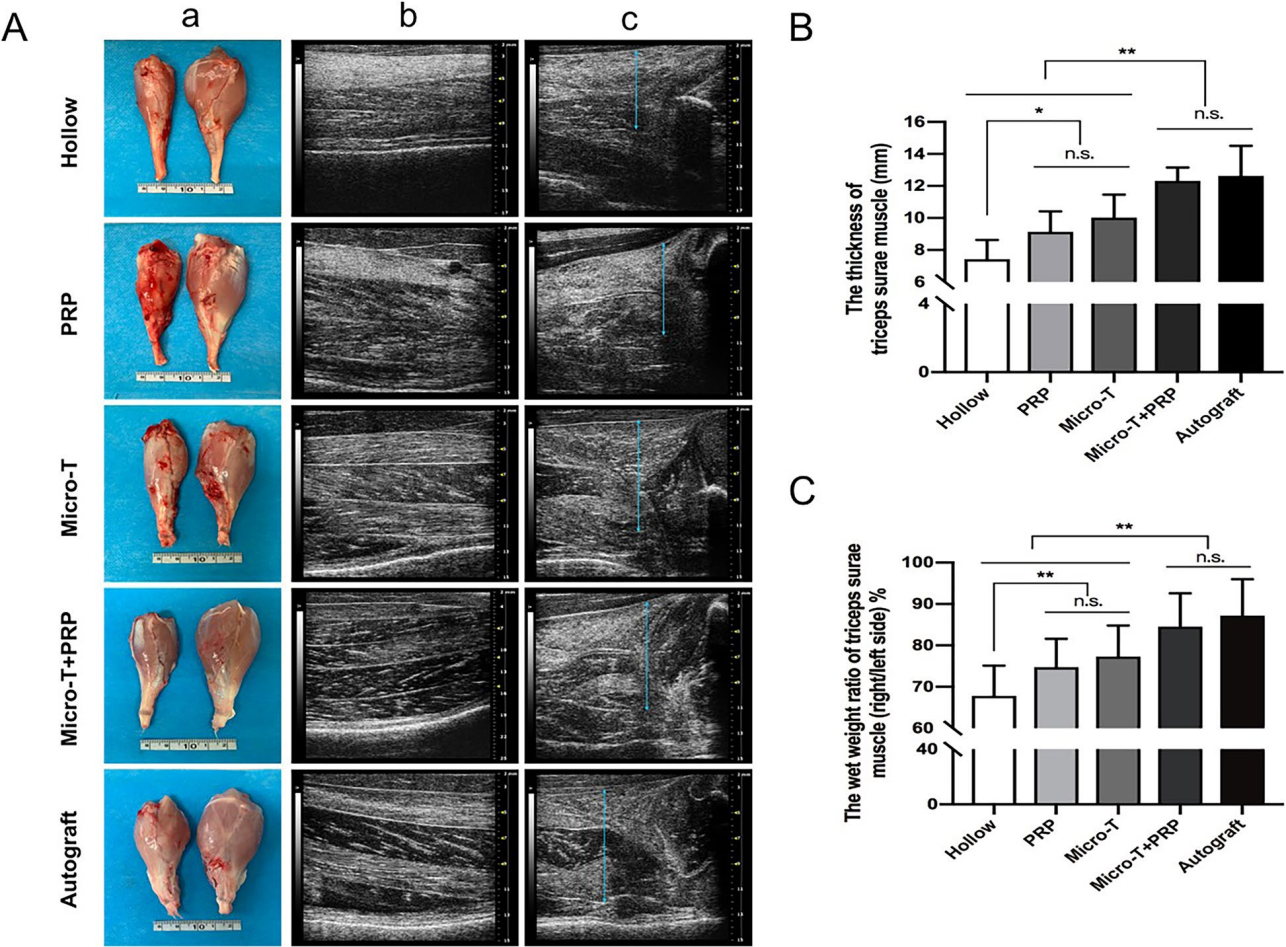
Macroscopic evaluation and high-frequency ultrasound evaluation of the targeted muscle. (A) Images of the targeted muscle. (a) The gross image; (b) The triceps surae muscle in the longitudinal section; (c) The thickness of the triceps surae muscle in the transverse scan. (B) Comparison of the thickness of triceps surae muscle in each group. (C) Analysis of the wet weight ratio of triceps surae muscle between groups. ^*^*P* < 0.05, ^^*P* < 0.01, and n.s. not significant. Micro-T microtissues, PRP platelet-rich plasma
